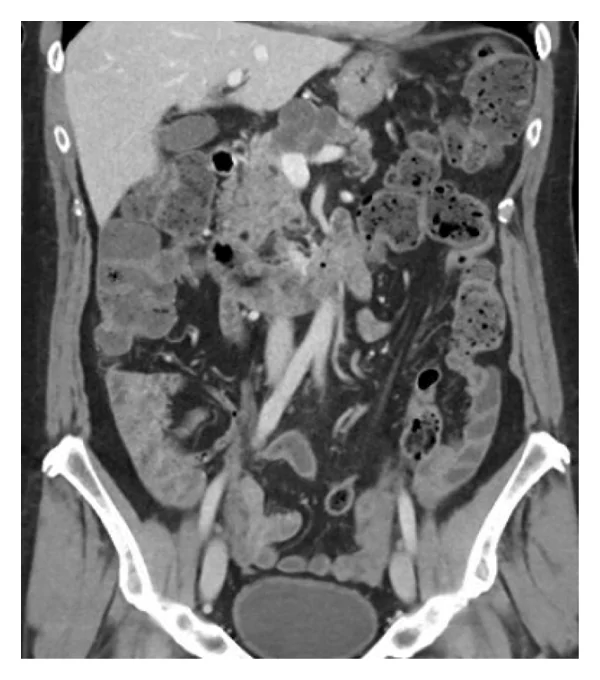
The patient’s CT in the coronal plane with a 4.9 × 4.7 cm complex, septated cystic pancreatic mass.
